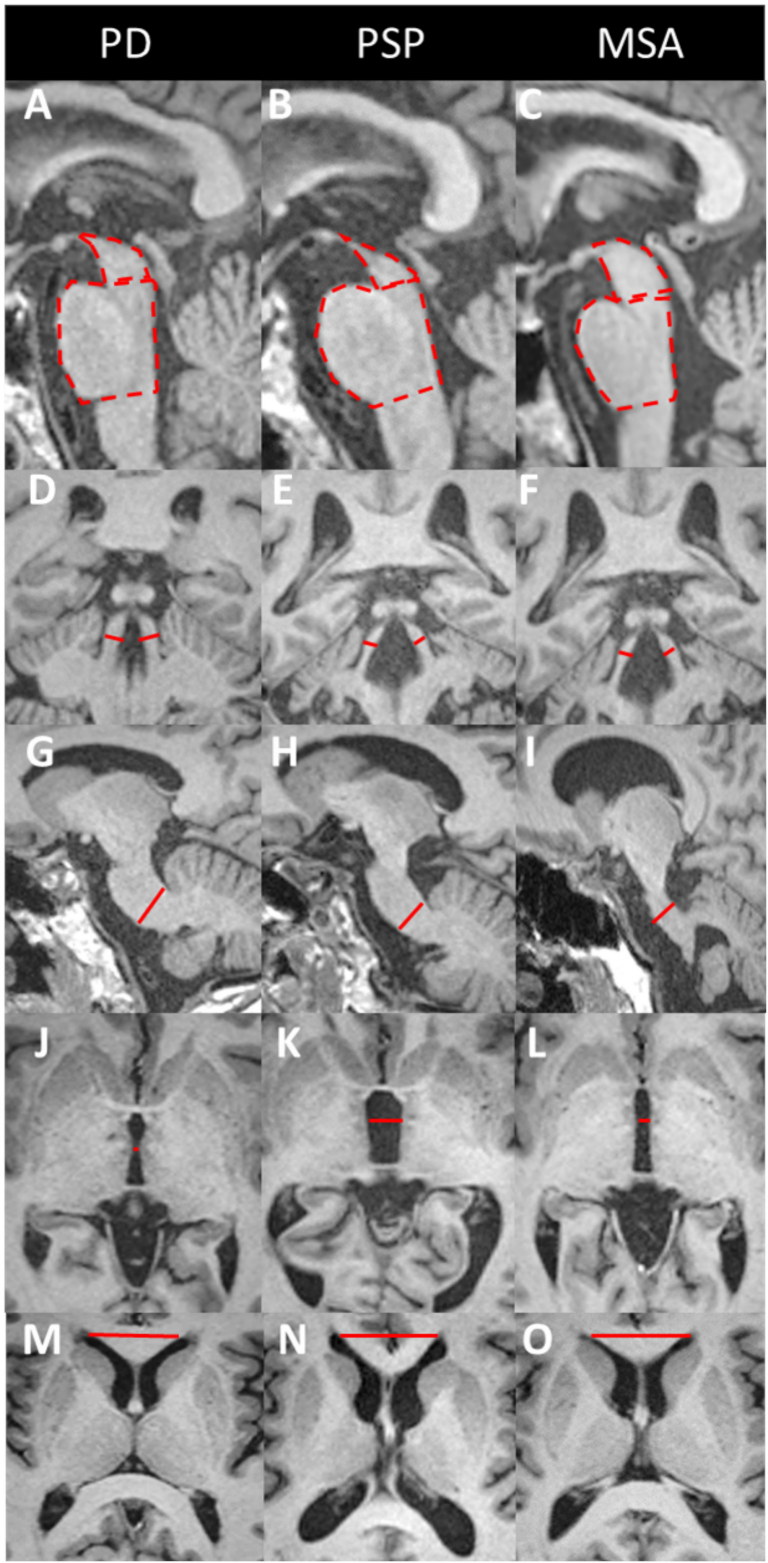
Midbrain to pons area ratio, Magnetic resonance Parkinsonism index (MRPI) and MRPI 2.0. Three-dimensional T1-weighted images in patients with PD (left), PSP (middle), and MSA (right). The MRPI combines measurements of the midsagittal surfaces of the midbrain (dashed line) and pons (dashed line) (A–C) and widths of the superior (red lines, D–F) and middle (red line, G–I) cerebellar peduncles [MRPI = (pons/midbrain) × (middle cerebellar peduncle/superior cerebellar peduncle)]. The limit between the midbrain and pons is defined by the line passing through the inferior border of the inferior colliculus and the pontomesencephalic sulcus. The inferior border of the pons is parallel to the previous one. The widths of the superior (D–F) and middle cerebellar peduncles (G–I) are measured in the parasagittal and coronal planes that best show the peduncles, respectively. The MRPI 2.0 is the product of the MRPI by the ratio of the width of the third ventricle (red line, J–L) to the largest left-to right width of the frontal horns of the lateral ventricles (red line, M–O) on axial views. For further details about the measurement technique [please refer to Quattrone et al. (46, 85)]. PSP patients show atrophy of the midbrain (B) and superior cerebellar peduncles (E) and enlargement of the third ventricle (K). In MSA patients, the atrophy is greater in the pons (C) and middle cerebellar peduncles (I), with an enlarged fourth ventricle. PD patients do not show any significant brainstem atrophy or MRPI changes. MSA, multiple system atrophy; PD, Parkinson's disease; PSP, progressive supranuclear palsy.
